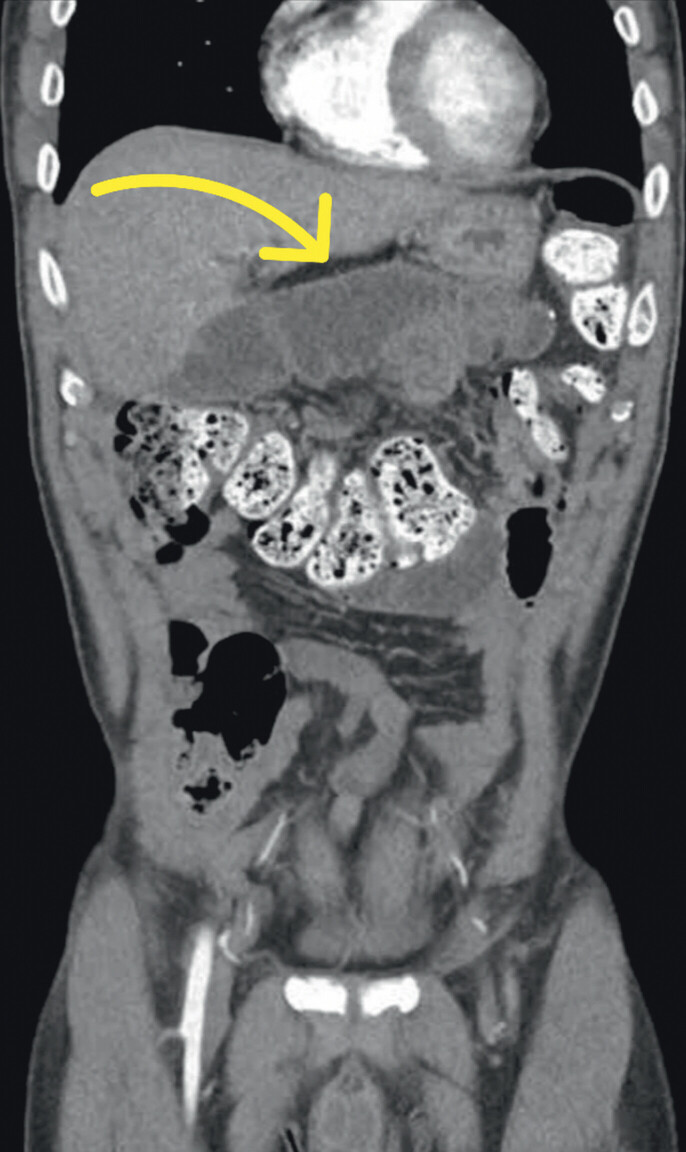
An index CT scan showing the dilated afferent limb, highlighted
